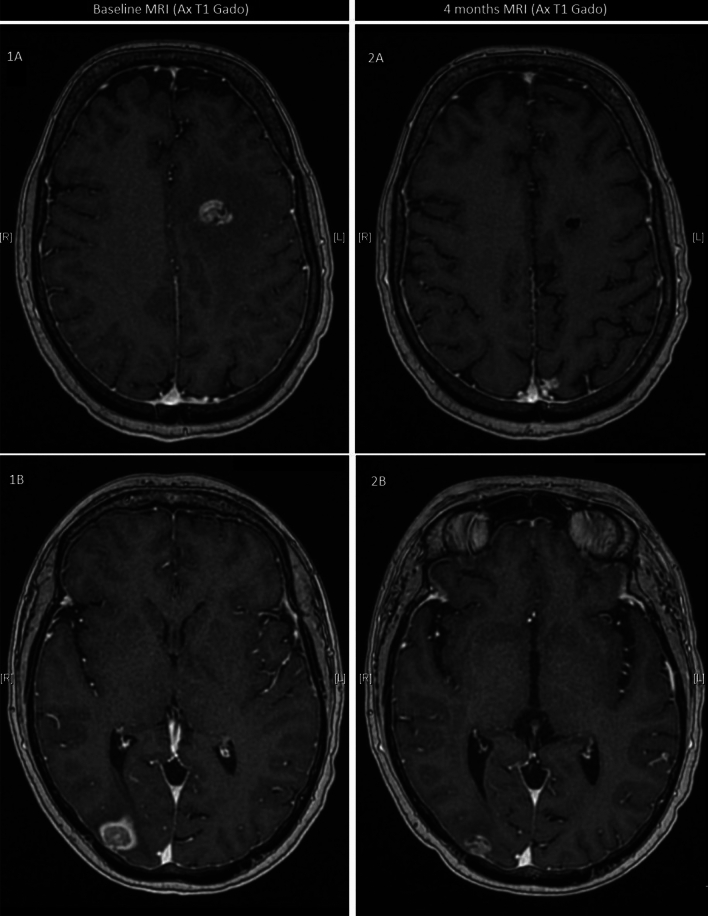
Best intracranial response with sacituzumab govitecan alone (without radiotherapy). Magnetic resonance imaging (MRI), T1 sequence with gadolinium injection. 1A/1B: target brain metastases before SG; 2A/2B: target brain metastases after 4 months with SG alone, without any radiation therapy
